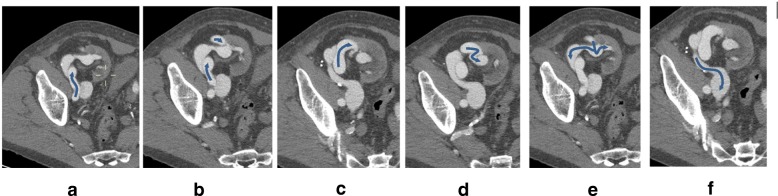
Series of CTA images demonstrating high flow arterio-venous fistula within the transplanted kidney. Block arrow demonstrating route of blood from transplant artery (images a-d), the AV fistula (image e) and the early filling of the transplant renal vein (image f)
